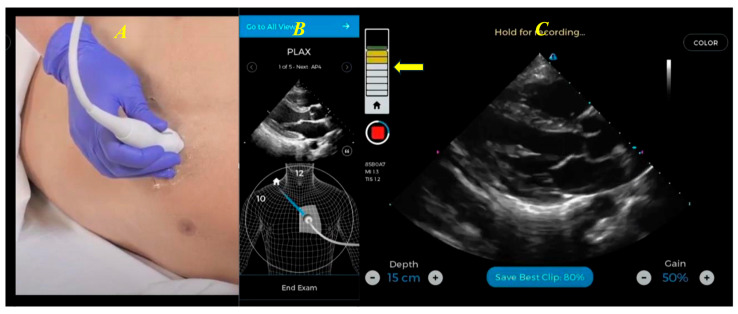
Training software for image acquisition, designed for cardiologists or non-imaging personnel (e.g., nurses, technicians). The transducer is positioned on the patient’s chest (A), while the software provides real-time guidance (instructions for movement, adjustment, or rotation of the probe) to the operator (B), in order to obtain the optimal parasternal long-axis view. The software uses a feedback bar (yellow arrow) where green indicates optimal positioning, and once achieved, the clip is automatically stored (C). Modified from Narang et al. [8].
